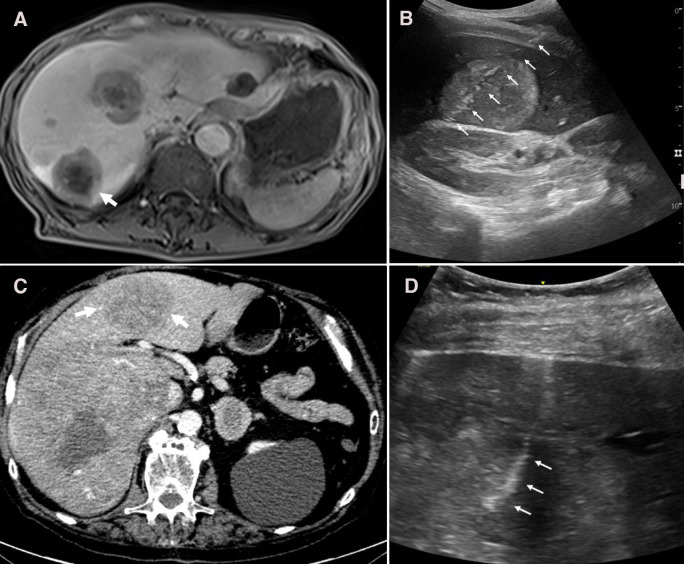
Cases #3 & 4, Panel (A) Case #3 pre-procedure post-contrast T1 MRI showing liver mass (white arrow). Panel (B) Case #3 post-biopsy and SinglePass device electrocautery needle path (white arrows). Panel (C) Case #4 pre-procedure contrast-enhanced CT imaging showing liver mass (white arrows). Panel (D) Case #4 post-biopsy and SinglePass device electrocautery ultrasound image showing needle tract (white arrows).
